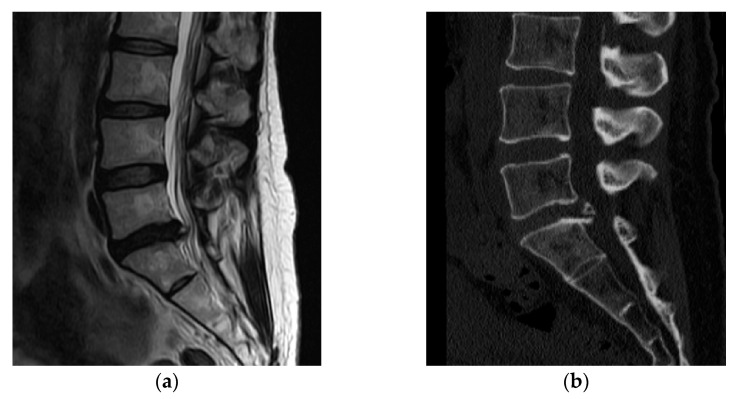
A 43-year-old woman presented with a radiating pain in her left lower extremity. (a) Magnetic resonance imaging (MRI) showed left central herniated disc at the L5/S1 level. (b) Computed tomography (CT) revealed a combined posterior ring apophysis fracture at the same level.
